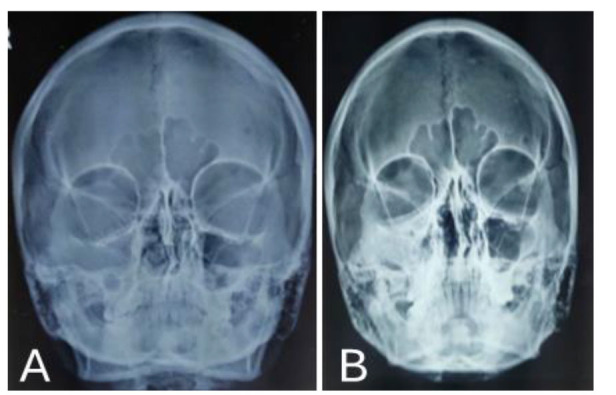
X-ray of paranasal sinuses before (A) and after (B) starting liposomal amphotericin B therapy
